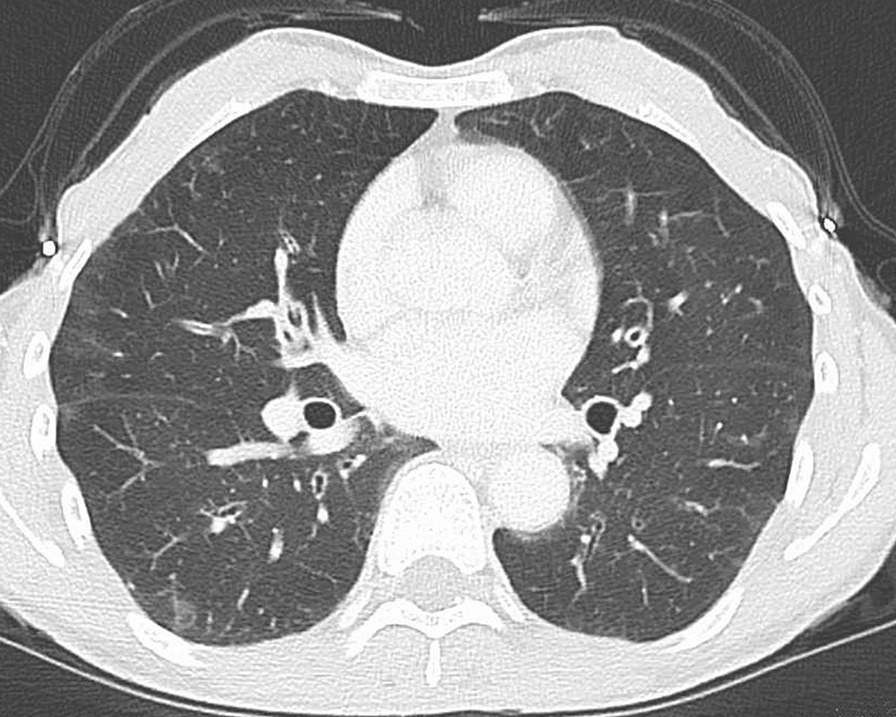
Bilateral patchy ground-glass opacity areas that were not distinguishable from the residual lesion of COVID-19 were observed on chest computed tomography
